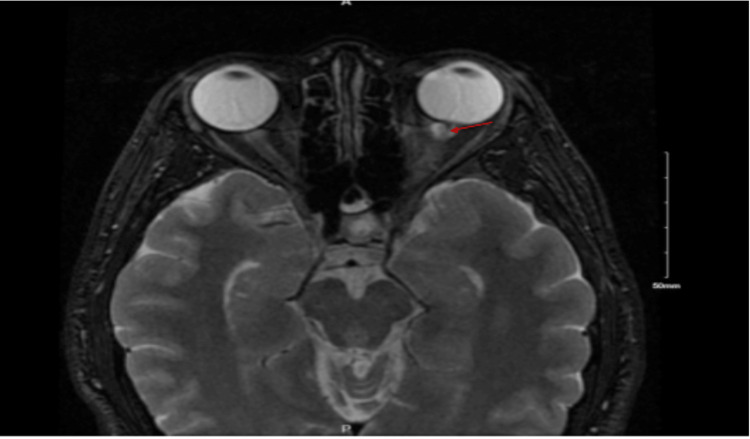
T1 fat-saturated MRI of the orbits demonstrating multifocal enhancement of the left optic nerve (red arrow) during acute presentation, consistent with optic neuritis.
